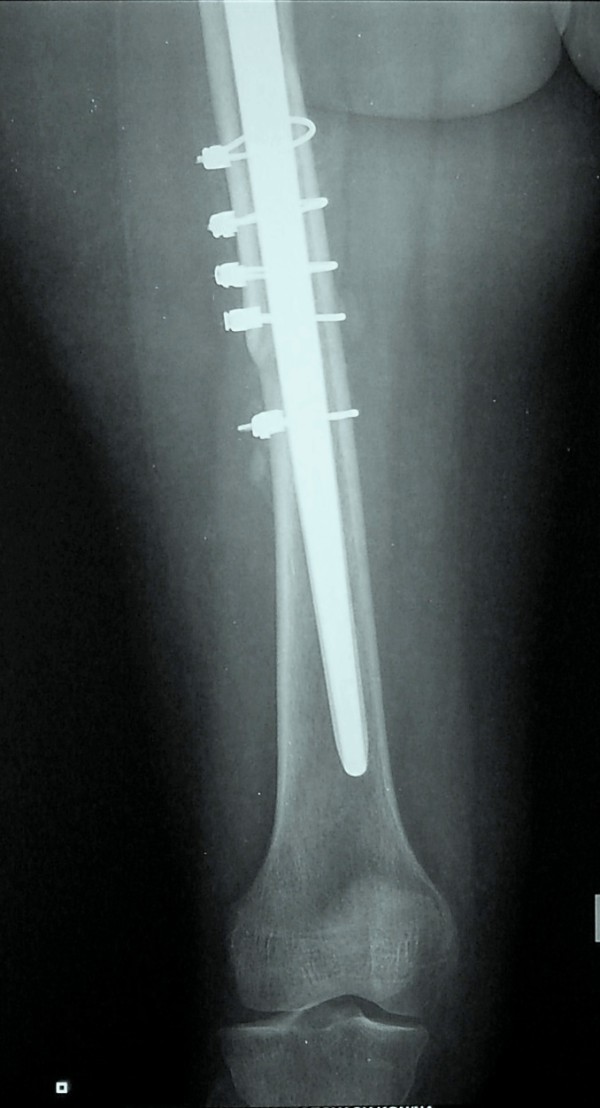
Distal anteroposterior X-ray at three postoperative months. The fracture has healed.
